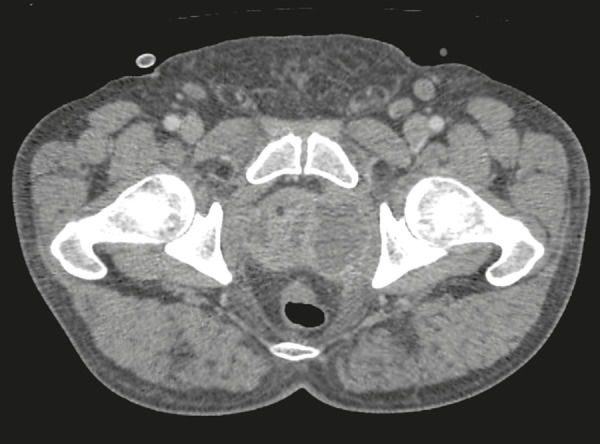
Axial contrast-enhanced computed tomography of the pelvis, showing a prostatic abscess.
